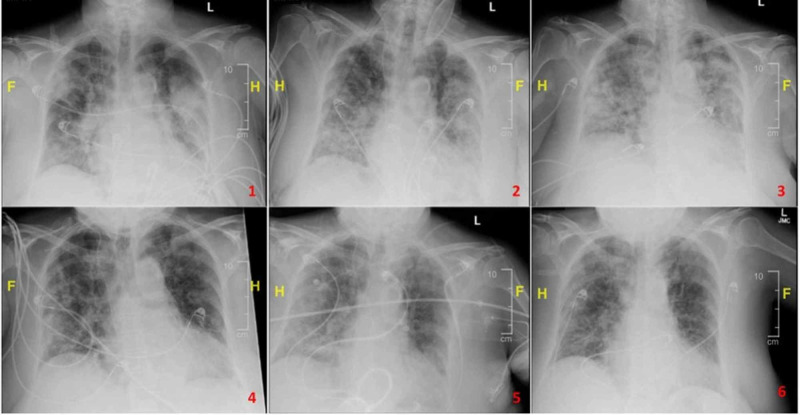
Serial portable chest X-rays demonstrating COVID-19 pneumonia lung-related changes 1: day 1 (admission); 2: day 3; 3: day 5; 4: day 7; 5: day 9; 6: day 11
